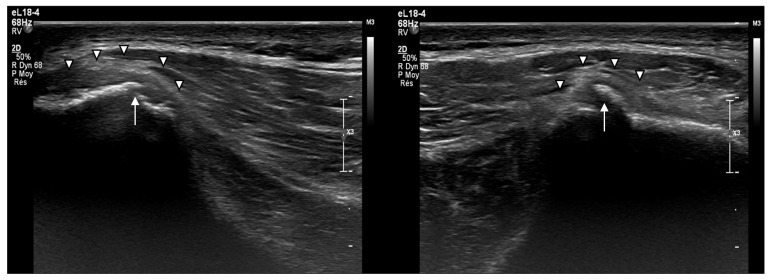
The seven-month follow-up ultrasound scans of the posterior iliac crest in Case 1: (left) the sagittal plane and (right) the transverse plane, showing the periosteal thickening (arrowhead) and calcification (arrow).
